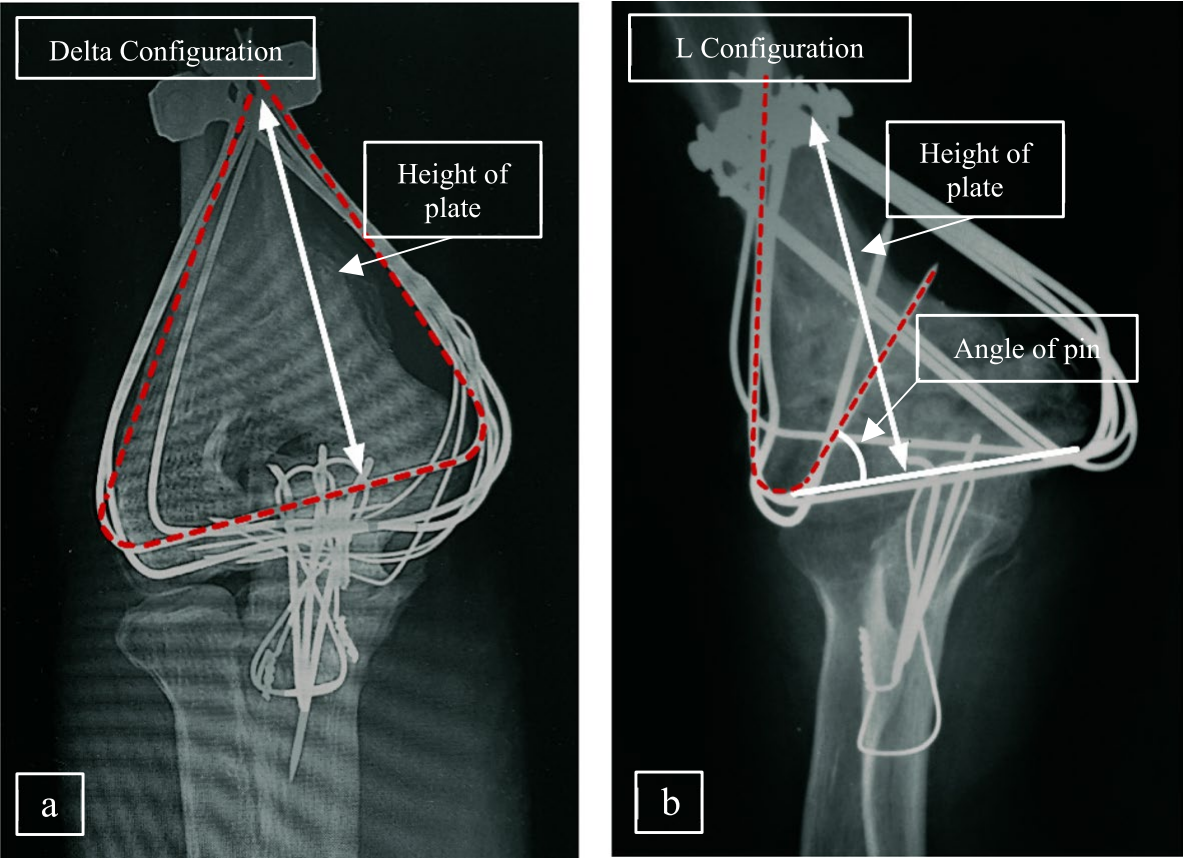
Anteroposterior radiograph images of (a) Delta and (b) L configurations.
